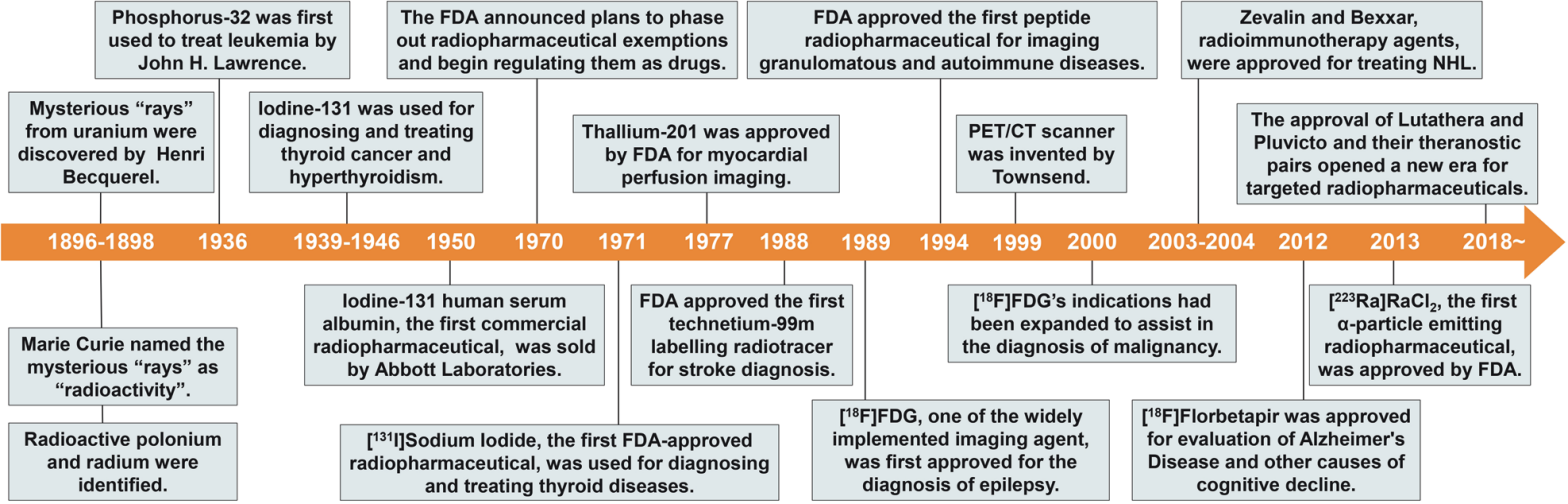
Overview of significant milestones and regulatory approvals for the discovery of radiopharmaceuticals. The journey began in 1896 with Henri Becquerel’s accidental discovery of “rays” emitted from uranium, a phenomenon later termed “radioactivity” by Marie Curie. Curie’s research further proceeded in the field by discovering the radioactive elements polonium and radium in 1898. In 1936, John H. Lawrence first used phosphorus-32 for the treatment of leukaemia which was the first example of the use of radionuclides in medicine. The commercial and regulatory landscape for radiopharmaceuticals began to take shape with the sale of the first commercial radiopharmaceutical, iodine-131 human serum albumin (RISA), by Abbott Laboratories. This regulatory evolution continued with the FDA’s decision to phase out the exemption for radiopharmaceuticals and regulate them as generic drugs. [^131^I]Sodium iodide was approved by FDA for treating thyroid disease. Technological and clinical advances further accelerated with the FDA’s approval of thallium-201 for myocardial perfusion imaging and the introduction of the first technetium-99m labelled radiopharmaceutical ([^99m^Tc]Tc-exametazime) for stroke diagnosis. [^18^F]FDG was first approved for identifying regions of abnormal glucose metabolism associated with foci of epileptic seizures in 1989. In 1999, the PET/CT scanner was invented by Dr. Townshend, who combined precise imaging with detailed anatomical information and improved diagnostic accuracy. On March 12, 2000, the FDA published a notice that expanded the approval of [^18^F]FDG for new indications. The therapeutic scope of radiopharmaceuticals was further expanded with the approval of the radioimmunotherapy drugs Zevalin and Bexxar for treating NHL. [^18^F]Florbetapir, the first Aβ-specific PET radiotracer approved by the FDA in 2012, was used for the evaluation of patients with cognitive impairment. Bayer’s [^223^Ra]RaCl_2_, the first α-particle radiopharmaceutical, markedly improved the treatment of metastatic cancers. Lutathera was the first FDA-approved radiopharmaceutical for targeted RPT in 2018. Another targeted RPT, Pluvicto, was approved for prostate cancer and achieved near-blockbuster status in 2023
